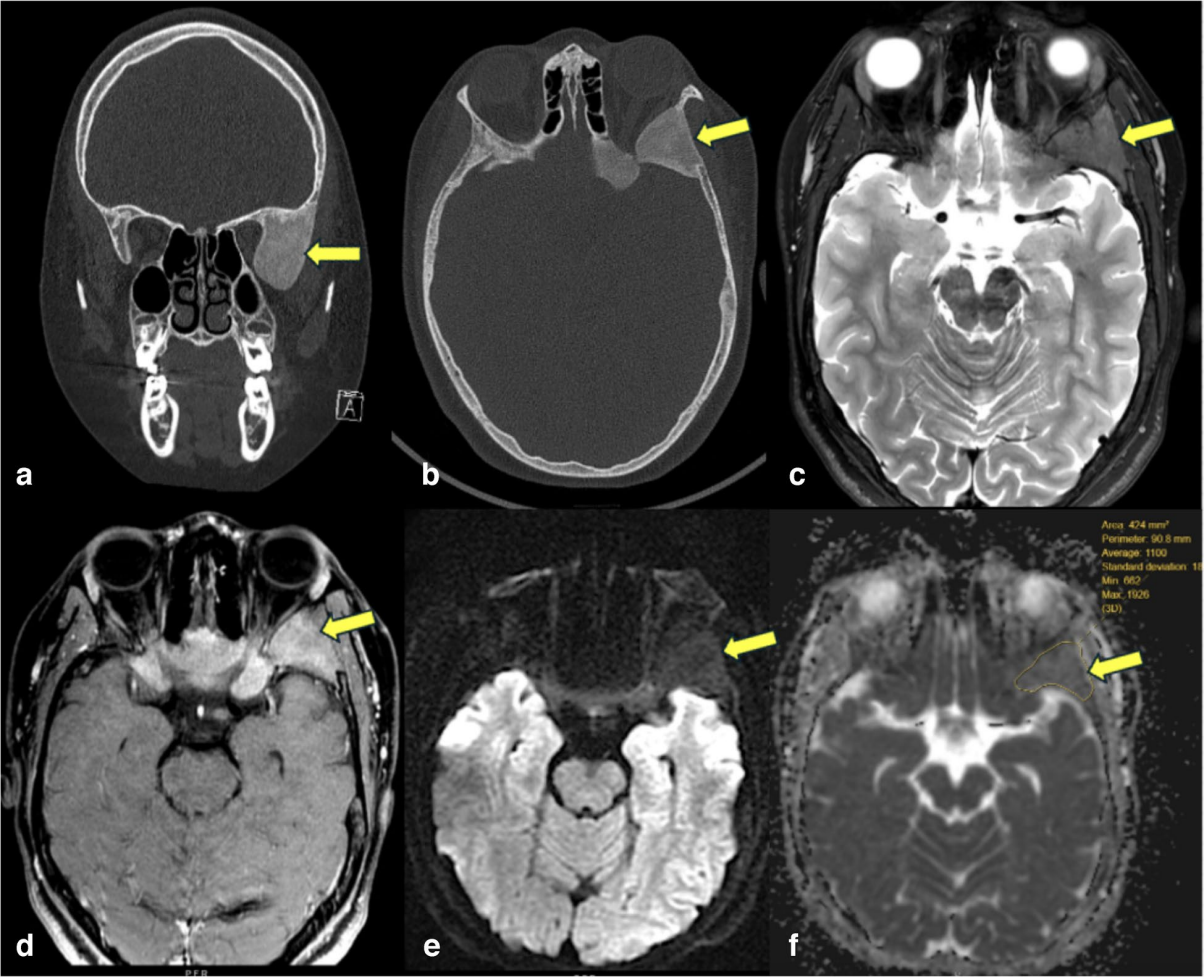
Fibrous dysplasia of the sphenoid bone in a 13-year-old male. a Coronal CT of the skull in bone window reveals an expansile lesion with ground glass attenuation (arrow) of the greater wing of the left sphenoid bone. b Axial CT image shows an expansile lesion with ground glass attenuation in the greater wing (arrow) and body of the left sphenoid. c Axial T2-weighted image demonstrates relatively low T2 signal of the lesion (arrow), consistent with fibrous stroma. d Axial post-contrast fat-suppressed T1-weighted image shows that the lesion enhances with contrast (arrow). e Axial DW-MRI reveals no restricted diffusion of the lesion (arrow). f Corresponding ADC map shows relatively low signal of the lesion, with ADCmean of 1.10 × 10^−3^ mm^2^/s ADCmin of 0.66 × 10^−3^ mm^2^/s, and ADCmax of 1.92 × 10^−3^ mm^2^/s
